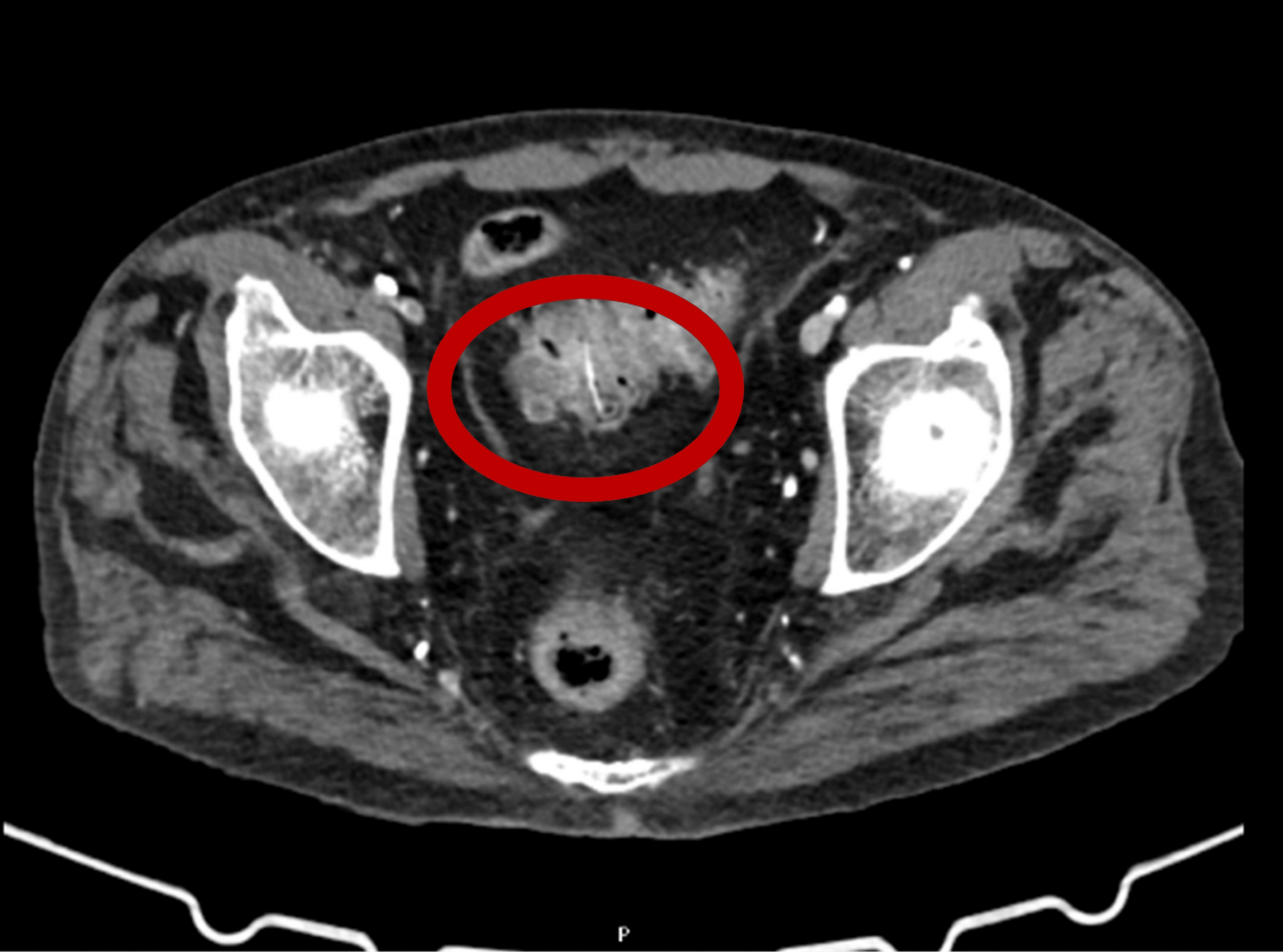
CT of the thorax, abdomen, and pelvis (CT-TAP) CT-TAP showing a faint, linear, dense image along the inferior border of the sigmoid colon wall (intraparietal) in its distal half, filiform in shape (longitudinal extension of 2.4 cm), of nonspecific nature, and not associated with the surrounding fluid collection.
